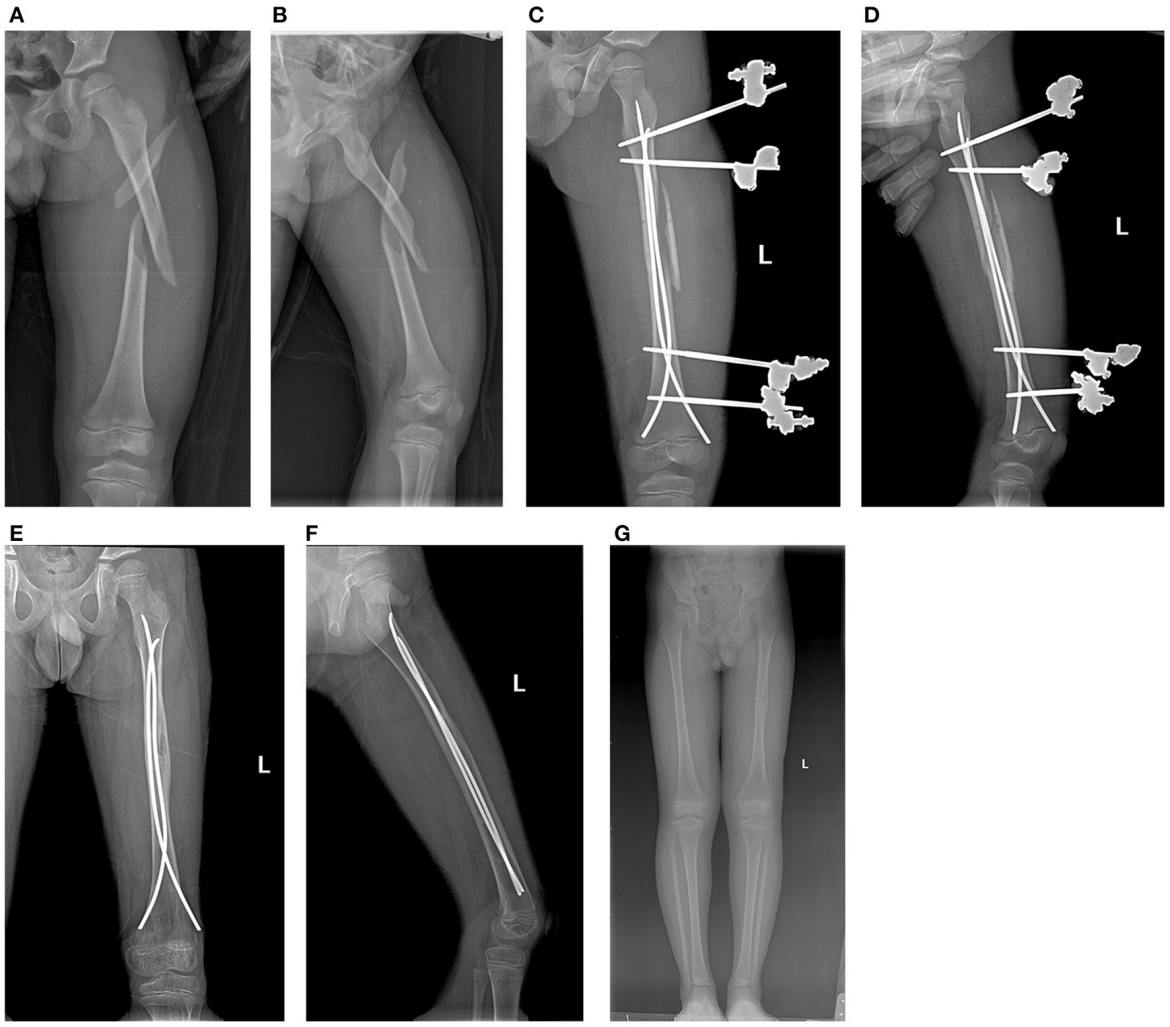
Case 5. (A,B) Comminuted type III femur fracture in an 8-year-old boy; (C,D) postoperative radiographs; (E,F) removal of EF; (G) final outcome.
